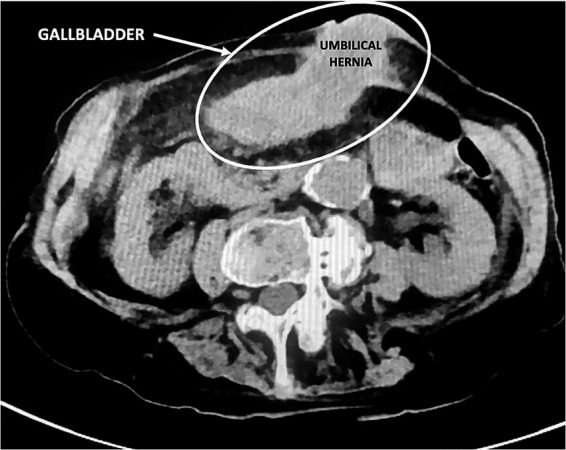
Preoperative CT scan clearly revealed what was later identified as the gallbladder within the umbilical hernia.
